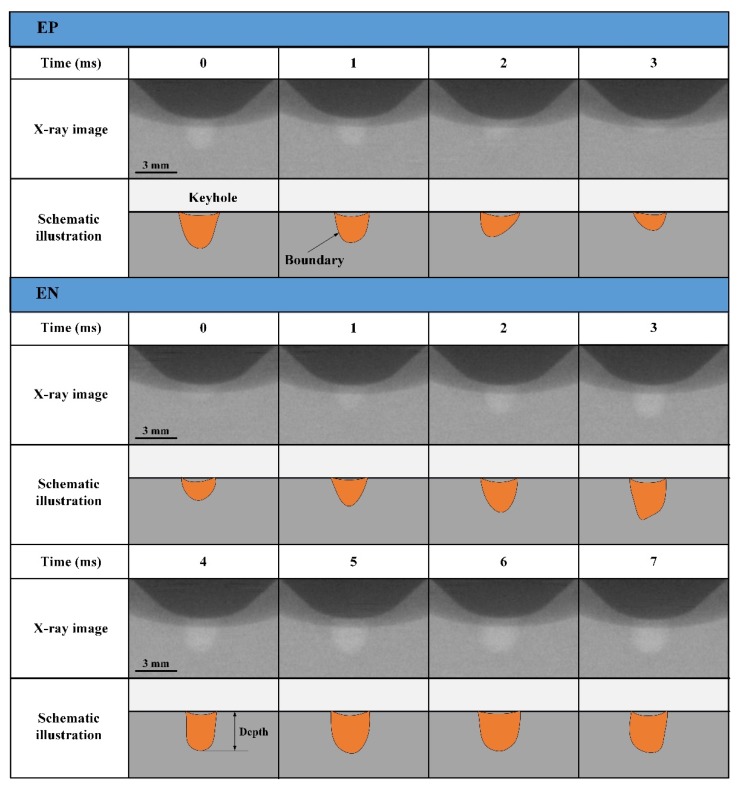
Dynamic characteristics of keyhole during the digging process shown by X-ray imaging technology.
